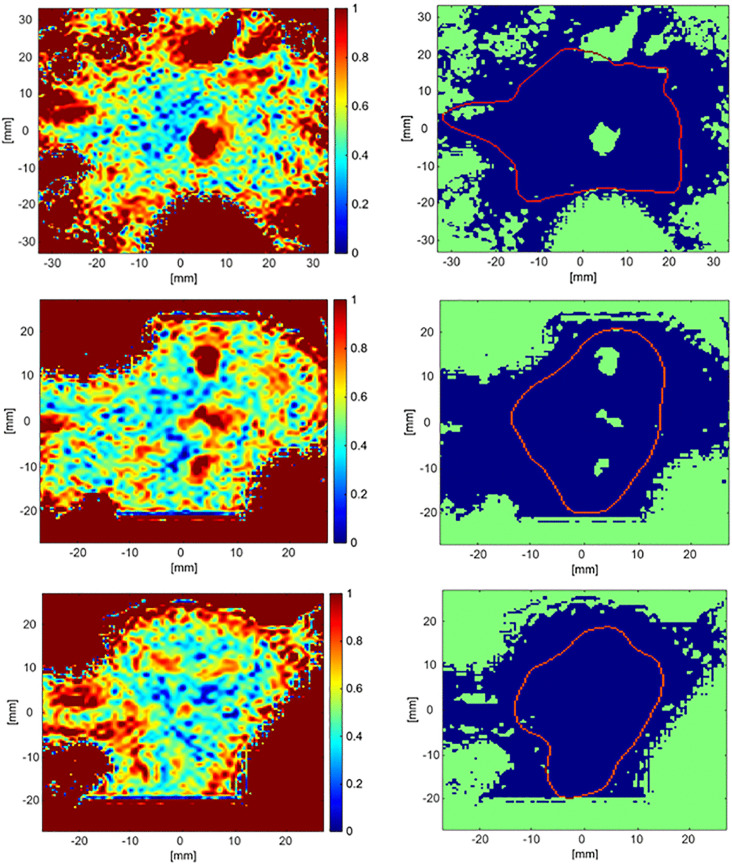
Three-dimensional gamma analysis comparing the TPS-calculated and R2-MRI–derived dose distributions using a gamma criterion of 3%/3 mm. The top, middle and bottom rows correspond to the central axial, coronal and sagittal slices, respectively. The left column displays the gamma index maps, while the right column shows the corresponding satisfactory agreement maps, with passing pixels (γ < 1) indicated in blue. In the satisfactory agreement maps, the red contour represents the 20% isodose line of the maximum dose (8 Gy) in the axial slice and the 50% isodose line in the coronal and sagittal slices. The 20% isodose contour is not shown in the coronal and sagittal views due to the limited MRI field of view, as discussed in the text.
